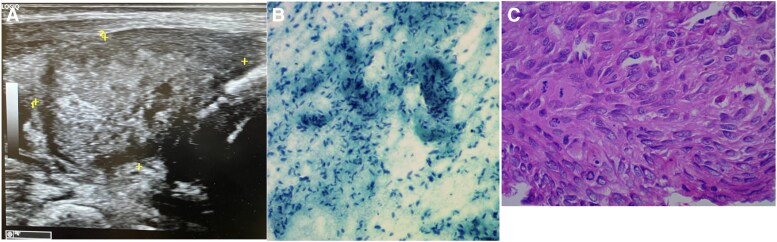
Case 3: (A) Ultrasound image of case showing American College of Radiology Thyroid Imaging Reporting and Data System Category 5 right thyroid nodule. (B) Fine-needle aspiration cytology of the thyroid nodule shows scattered spindle cells. They have oval pleomorphic nuclei with scanty cytoplasm. Few mitotic figures are seen, and no definite follicular cells are seen. (C) Biopsy from a muscle deposit showed spindle-shaped tumor cells arranged in fascicles, with moderate nuclear atypia, eosinophilic cytoplasm, and occasional giant cells, including atypical forms.
